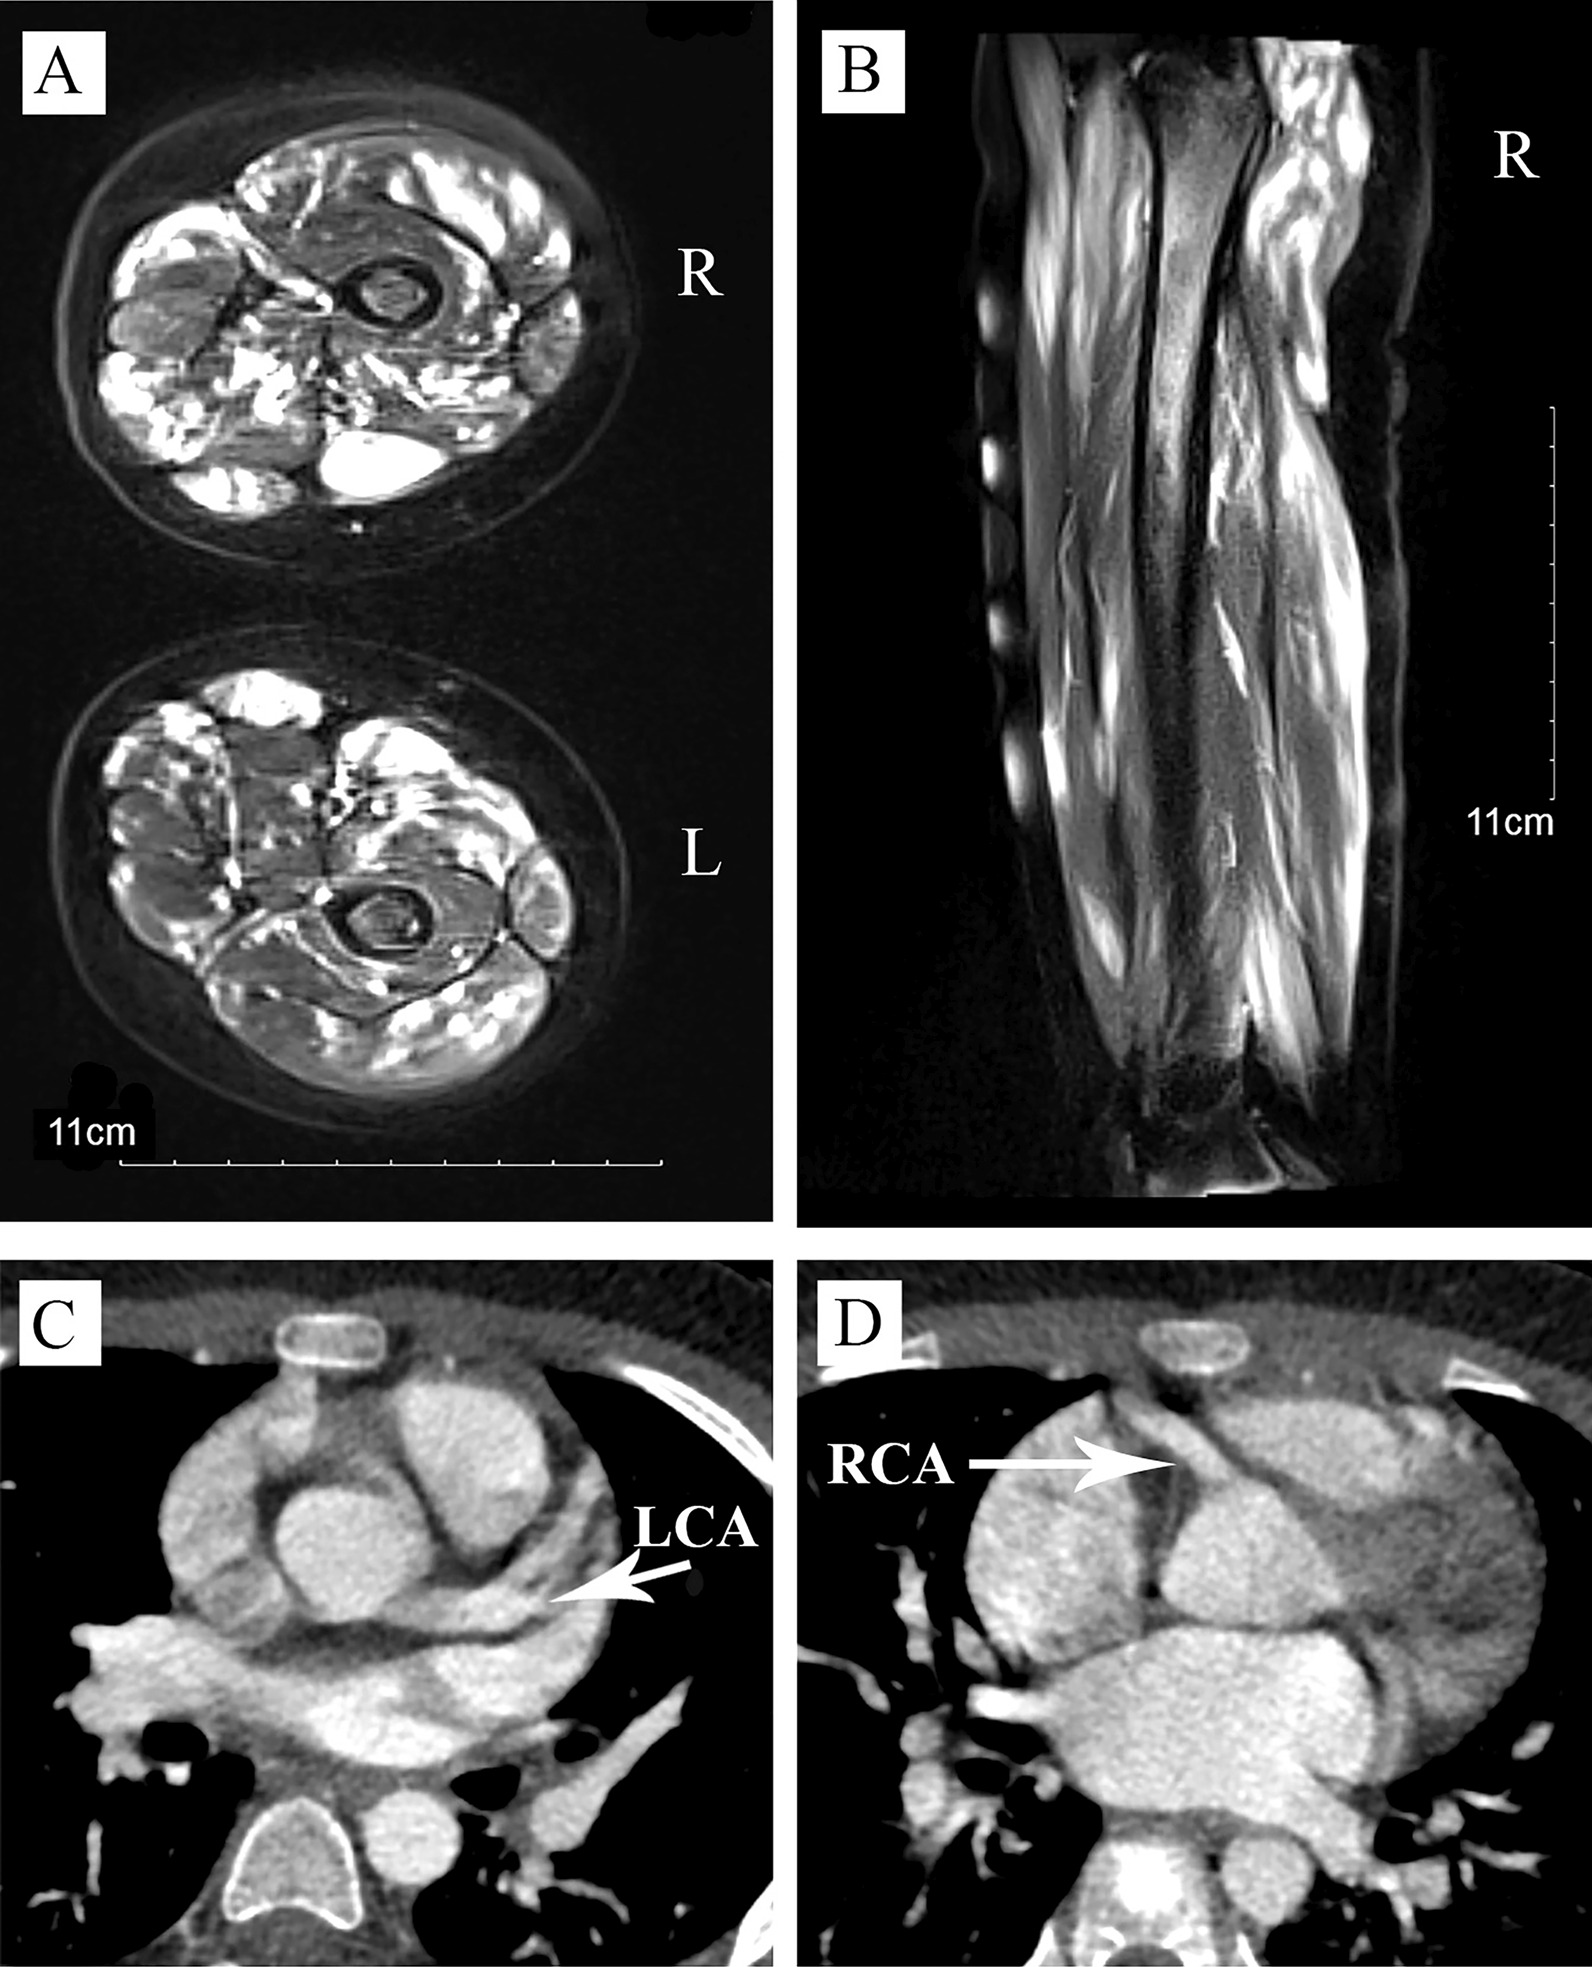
Imaging findings. The axial (A, right and left thighs) and sagittal (B, right thigh) T2-weighted magnetic resonance imaging (MRI) showed spotty high-intensity signal in thigh muscles such as adductor muscles, and femoral quadriceps muscles indicating muscle inflammation. Contrast-enhanced computed tomography angiography (CTA) showed left C and right D coronary artery dilations. *LCA *left coronary artery, *RCA *right coronary artery
